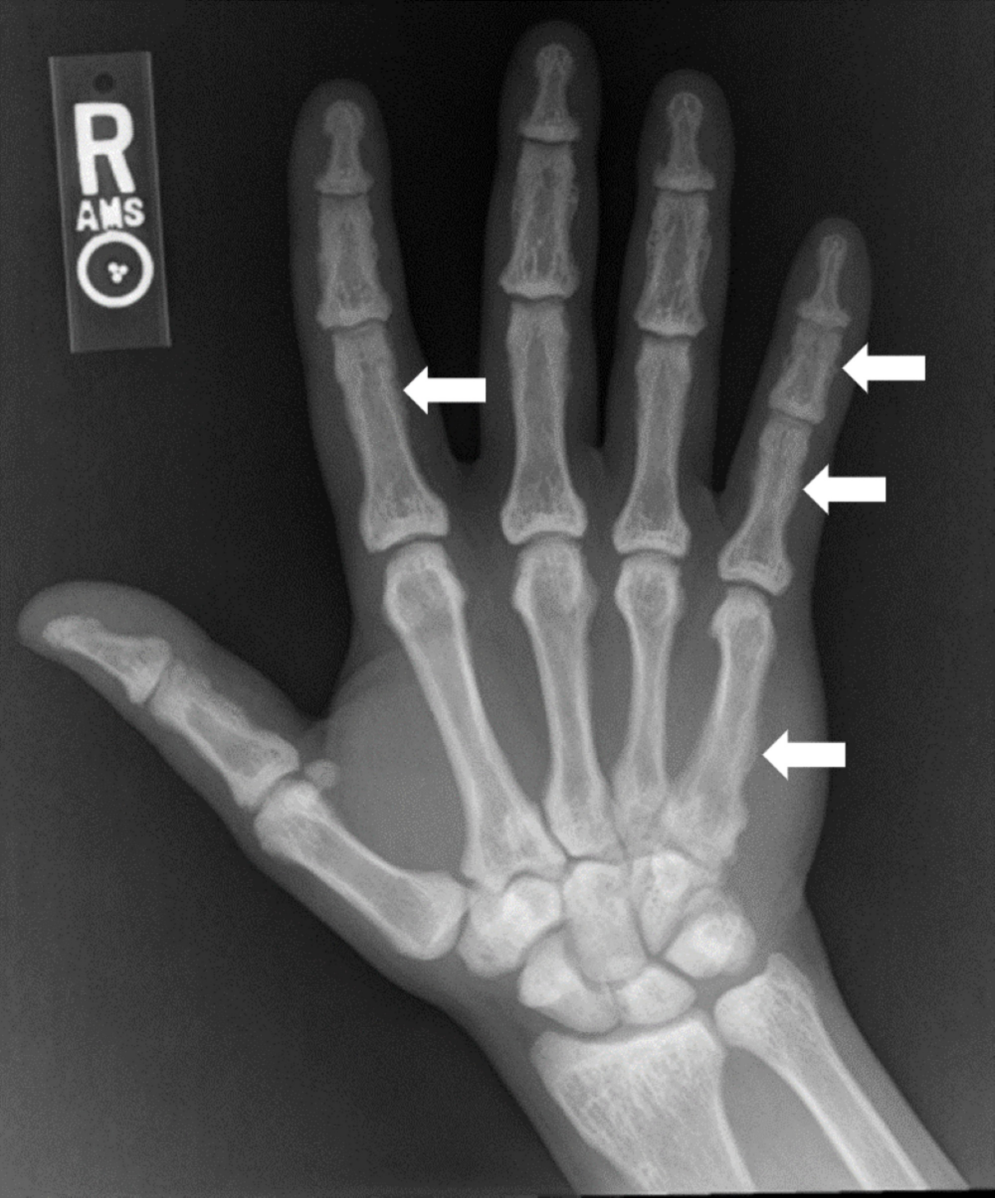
Right hand/wrist radiograph Diffuse sclerosis with multiple areas of indistinct cortex/periosteal reaction, most prominently involving the 2nd and 5th digits and 5th metacarpal.
